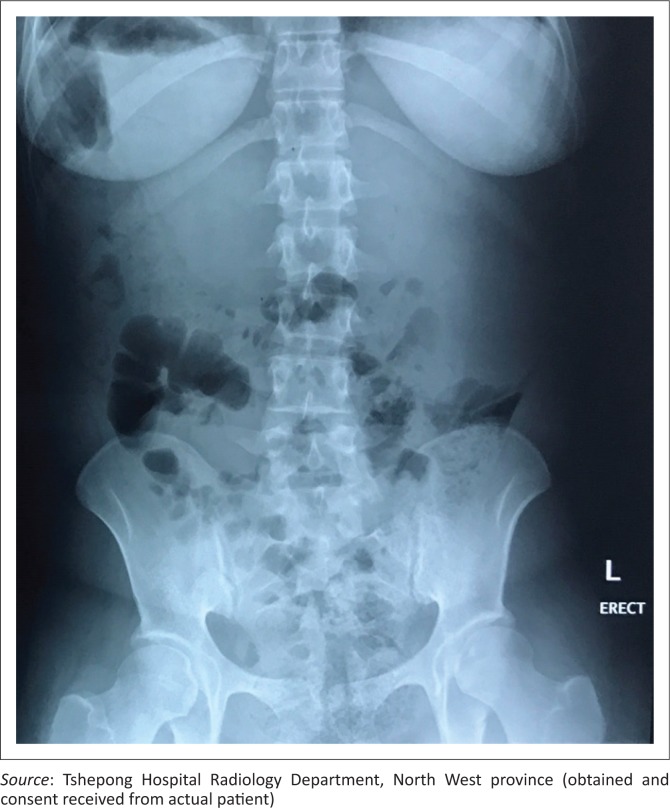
Erect abdominal radiograph demonstrating fullness of bilateral renal fossae with obscuration of the psoas outline suggestive of renal masses. Associated mass effect evidenced by inferior displacement of adjacent bowel loops.
